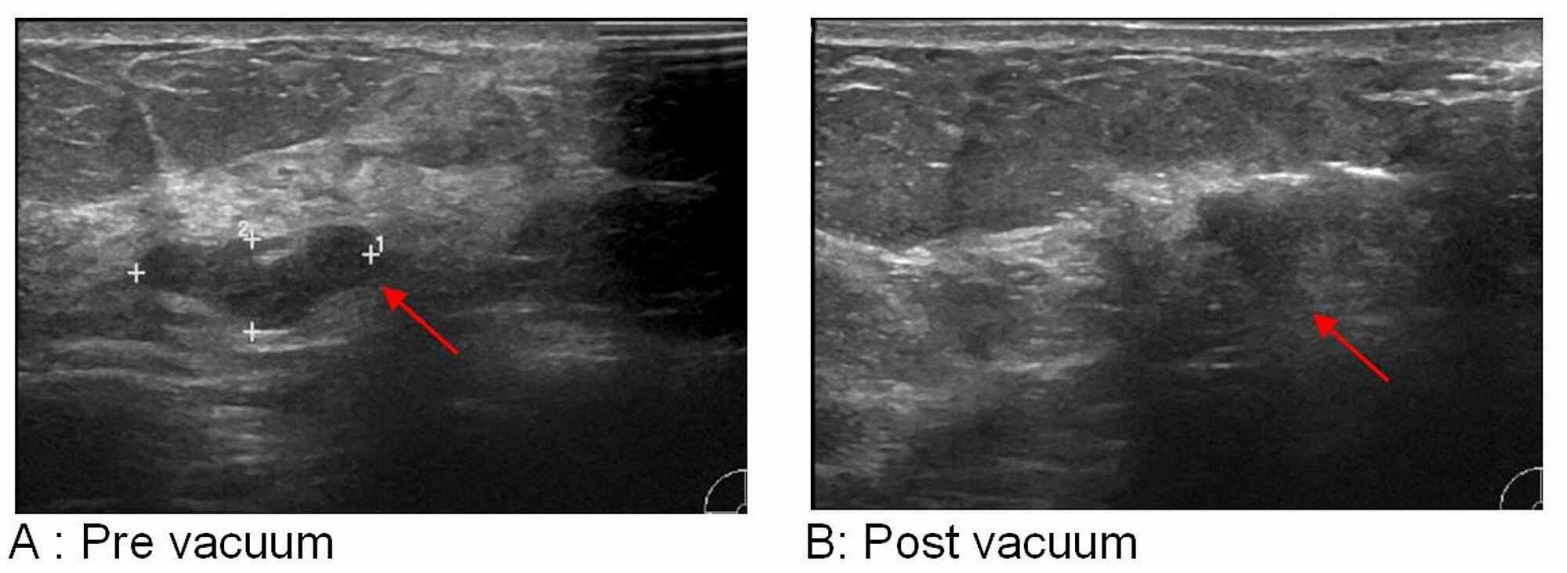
Left breast ultrasound scan of a 9 o’clock-located 16-mm fibroadenoma with LCIS in a 60-year-old female patient LCIS: lobular carcinoma in situ
